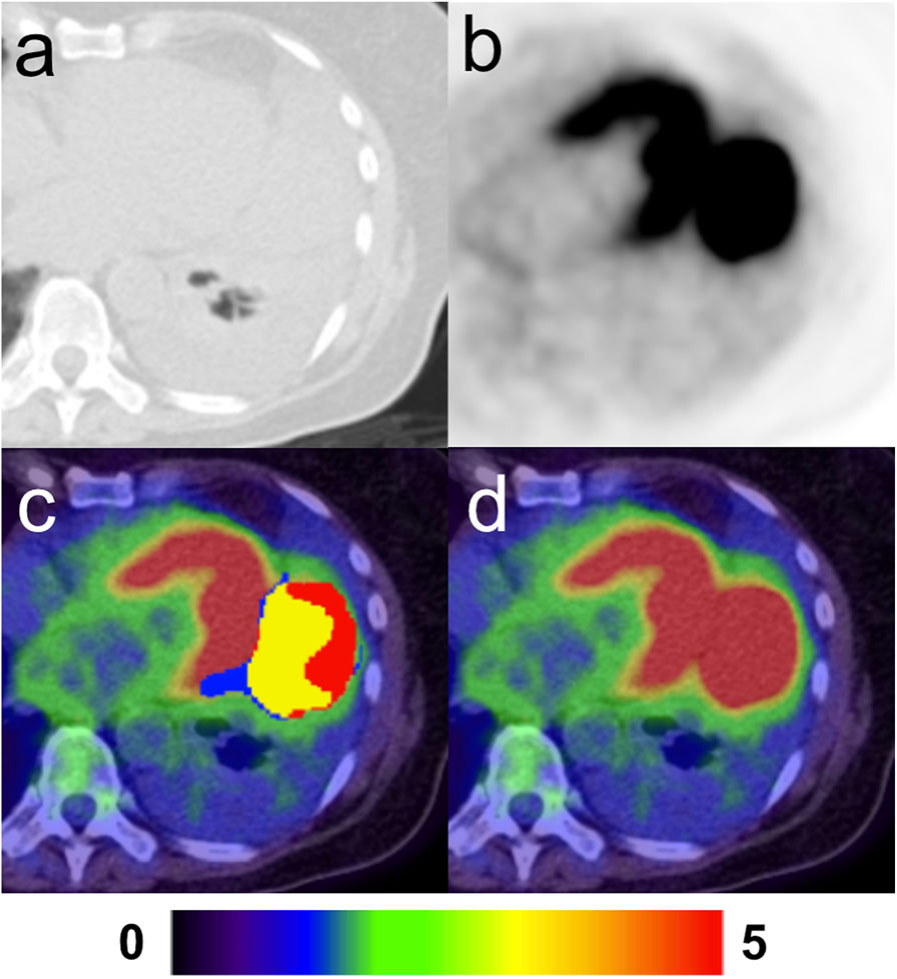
Large lesion in the left lung adjacent to the heart. a Axial CT with lung window. b Axial PET. c Fused axial PET-CT with overlaying segmentations; manual only (red), AI-only (blue) and manual + AI (yellow). d Fused axial PET-CT
